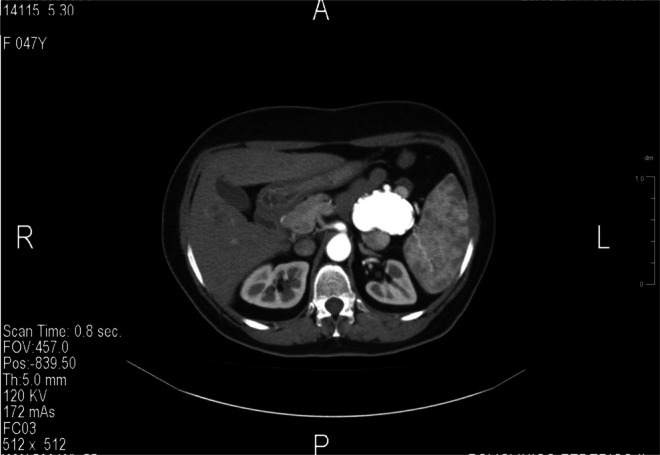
Computed tomography scan of the third lateral splenic artery aneurysm.
